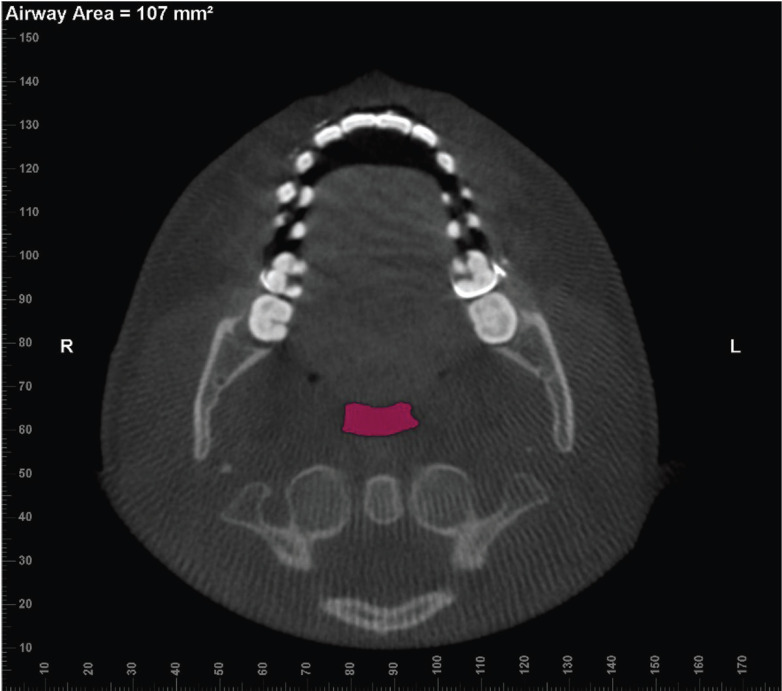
Axial reconstruction of CBCT image indicating the minimum cross-sectional axial area (CSA).
